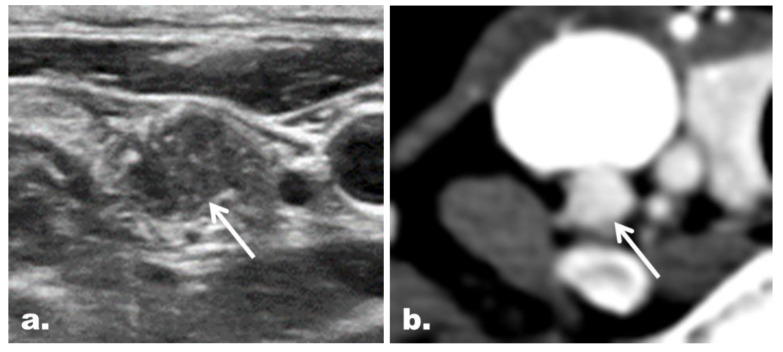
Representative case of a concordant suspicious LN with the final diagnosis of metastasis in a 41-year-old woman with PTC. (a) The gray scale US image depicts a round LN (arrow) with microcalcifications at the right neck level IV. The LN was classified as a suspicious LN on US. (b) The LN (arrow) showed diffuse strong enhancement on CT and thus was also classified as a suspicious LN on CT.
